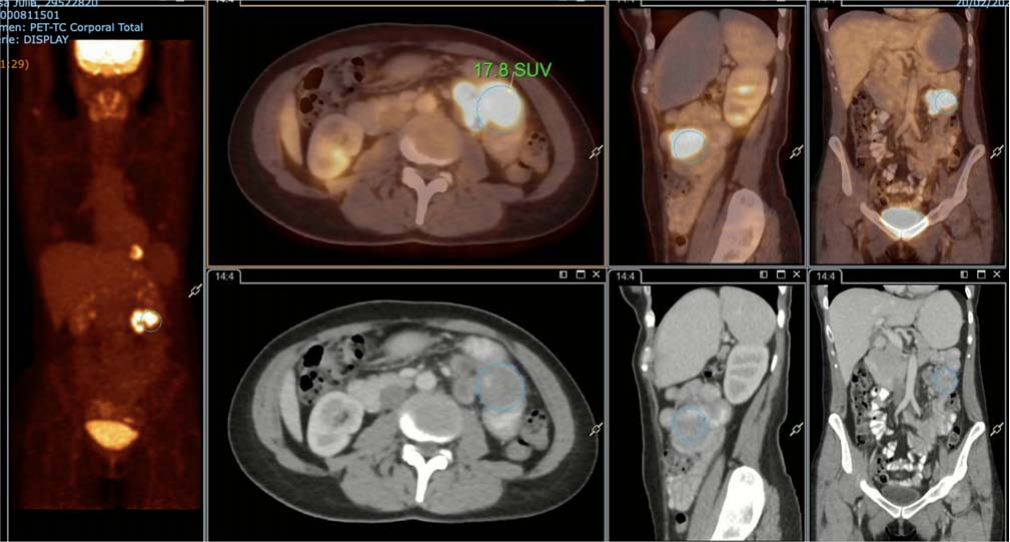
PET/CT showing jejunal wall thickening with increased uptake (SUVmax 17.8).
